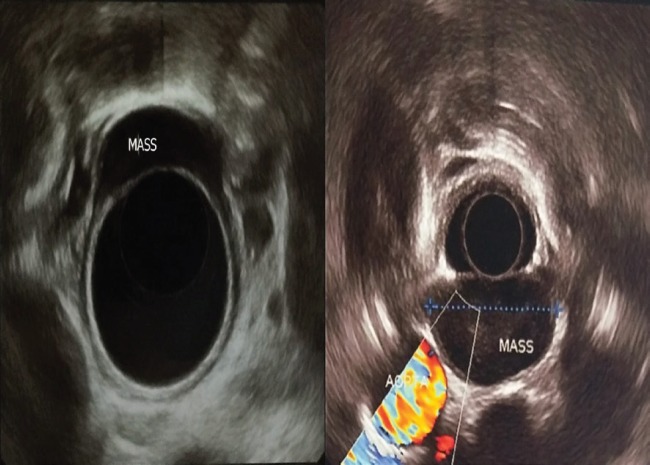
Endoscopic ultrasonography of case 1 (left) and case 2 (right). Left, 3.2 cm, hypoechoic, well capsulated, slightly heterogeneous mass from muscularis propria; Right, 1 cm, hypoechoic, and well capsulated, heterogeneous mass from muscularis propria (Figure 4, right).
